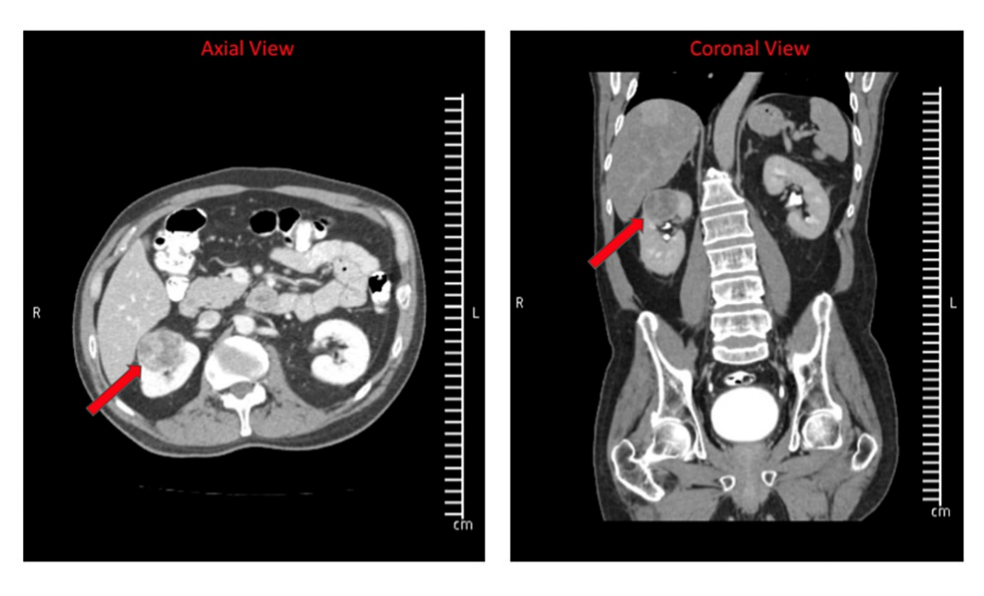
Axial and Coronal Computed Tomography Images of the Right Upper Pole Renal Mass (Arrows)
